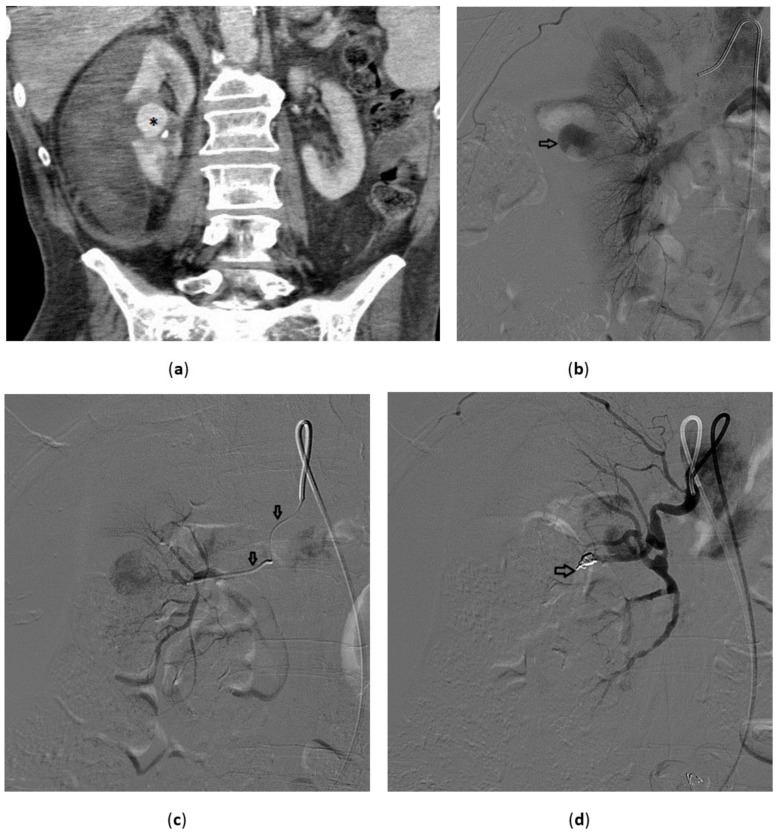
Renal pseudoaneurysm 79-year-old male with hematuria; previous attempt to insert a percutaneous nephrostomy on the right kidney. (a) Contrast enhanced CT: hyperdense round lesion (*) in the middle of the right kidney compatible with pseudoaneurysm with a large perirenal hematoma; (b) Right renal angiogram confirms the lesion (arrow). (c) A microcatheter was advanced in the feeding artery (arrows) and the visualization of the pseudoaneurysm after contrast media injection confirmed the correct position. (d) Two microcoils were deployed (arrow) and the pseudoaneurysm was no longer visible.
